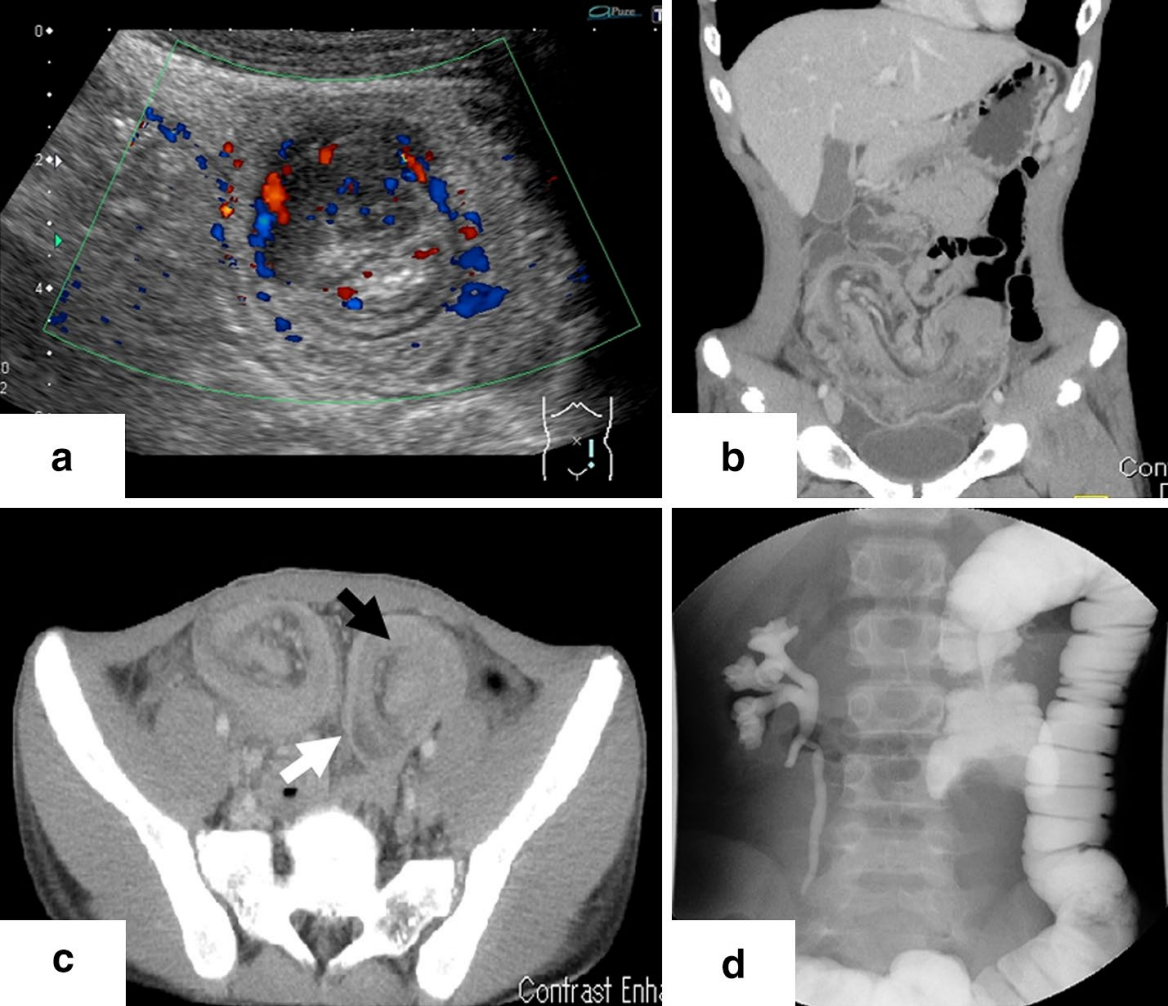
Preoperative images. a Ultrasonography showed the “target sign” in the lower abdomen. b Enhanced CT revealed ileocolic intussusception and stretched mesenteric vessels. c The outer wall of the area of intussusception consisted of an edematous ileum (inner layer; *black arrow*) and dilated colon (outer layer; *white arrow*). d An enema examination showed “crab’s claw sign” in the transverse colon.
